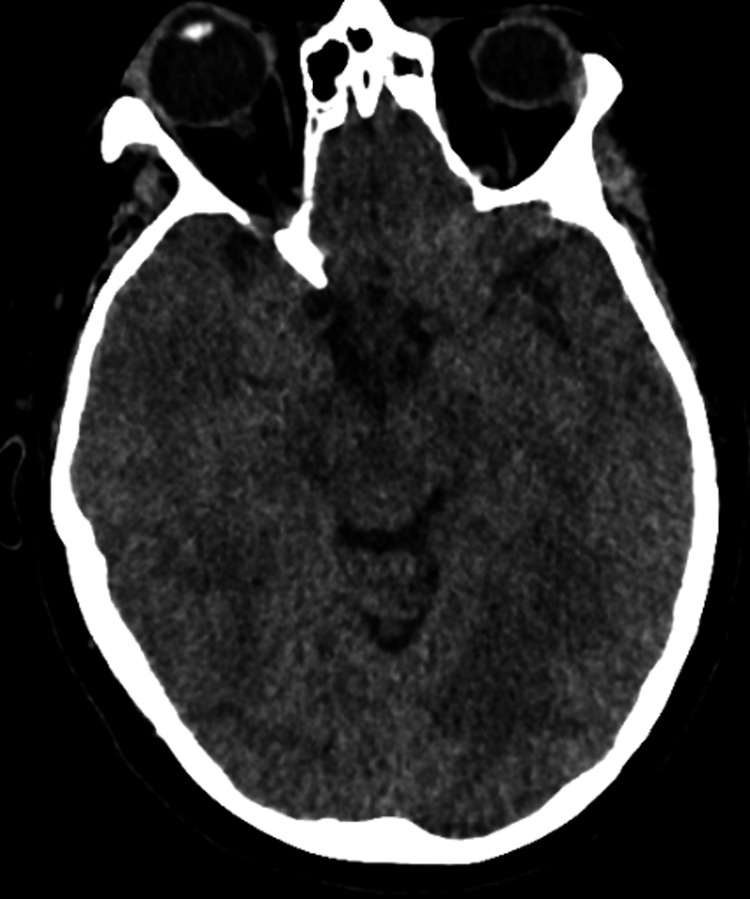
Urgent non-contrast CT Head (axial) obtained during the episode of hypertensive crisis and bradycardia, demonstrates marked effacement of extra-axial CSF spaces consistent with raised ICP. There is no evidence of transtentorial or tonsillar herniation, and basal cisterns remain patent.
